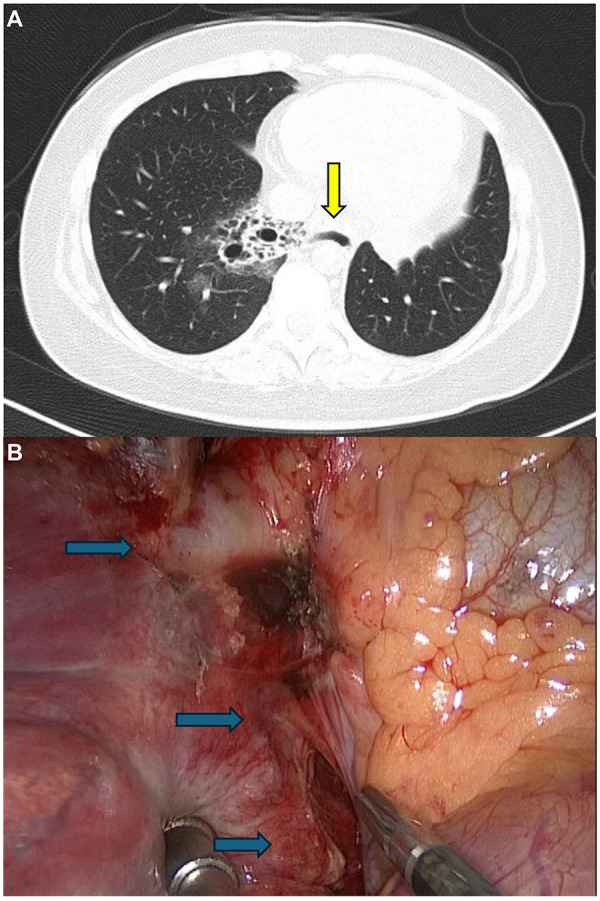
Inter-stage main radiologic evaluation and intraoperative surgical view. (A) Follow-up contrast-enhanced CT before the second-stage surgery (March 2025) showing the blind end of the left fistulous tract (indicated by arrow). (B) Intraoperative view during the right lower lobectomy (cranial to caudal perspective). The arrows sequentially demonstrate the right inferior pulmonary vein, the communicating sinus tract, and the right anomalous systemic artery.
